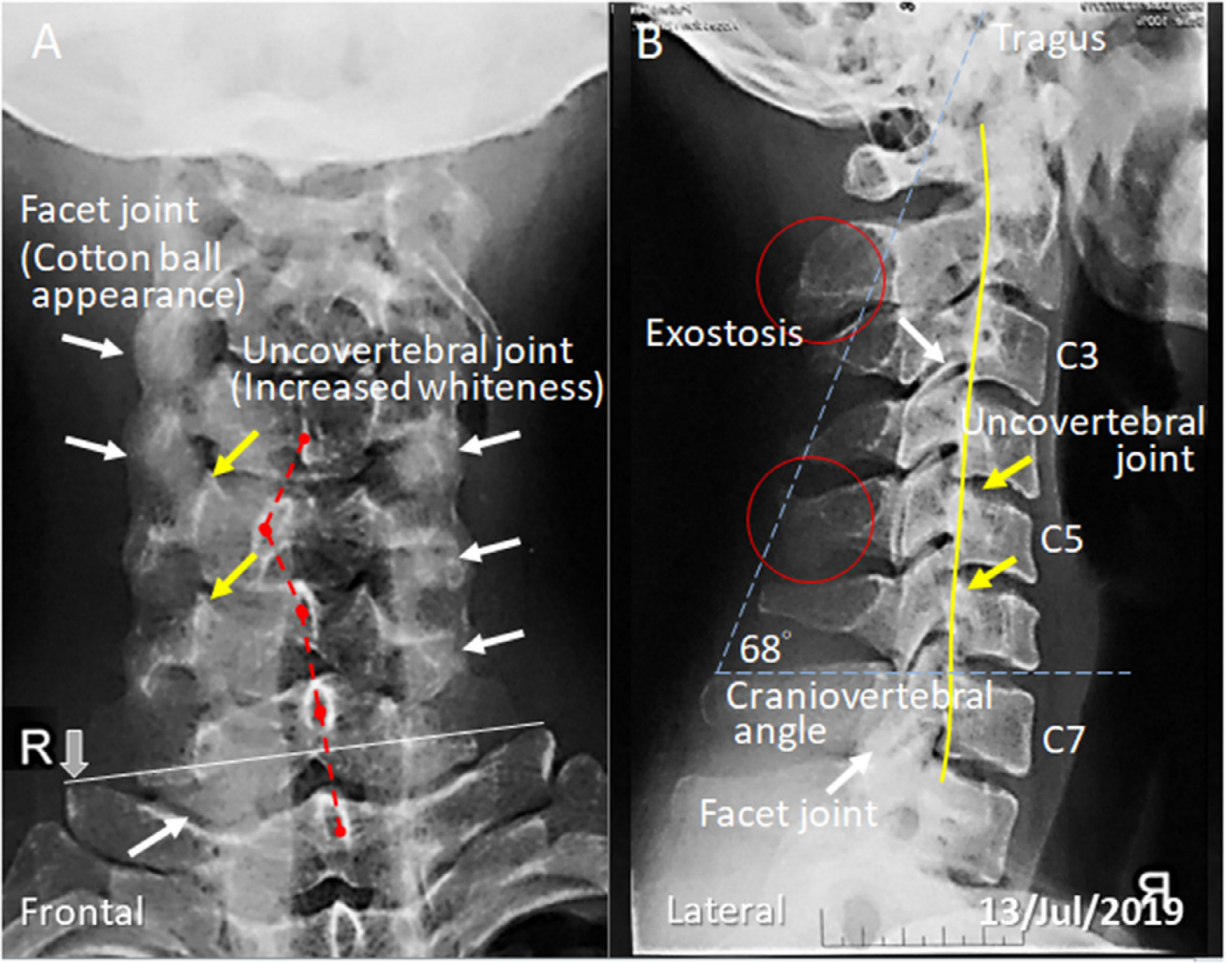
Cervical radiographs taken one year before chiropractic attention. (A) Frontal cervical X-ray shows a tilt to the right and degenerative hypertrophy of the facets (white arrows) and uncovertebral joint (yellow arrows). Dashed red line highlights malalignment of the C-5 spinous process, indicative of a vertebral rotation. (B) Lateral cervical x-rays revealed a loss of cervical lordosis (yellow line highlights posterior vertebral body margins). Global cervical curve is 0°, using the posterior vertebral body of C2 and the posterior vertebral body of C7. The exostoses (red circles) in the spinous process interspaces indicate a chronic injury of the nuchal osteo-ligamentous attachment. The craniovertebral angle is 68°. An angle less than 50° will be defined as forward head posture.
